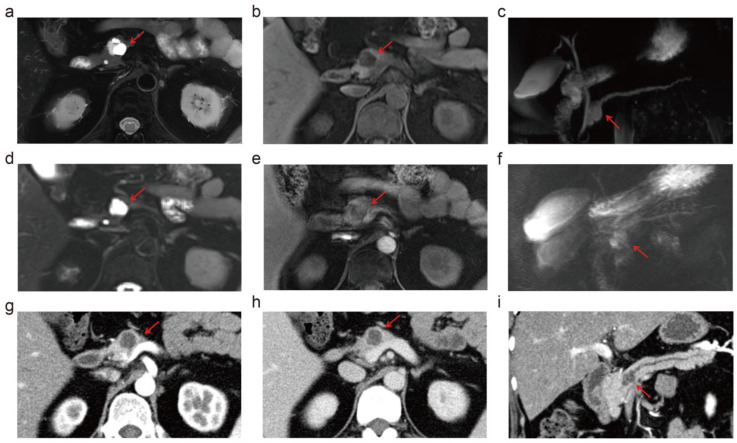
The images from 5.0 T MRI, 3.0 T MRI, and MDCT of a 56-year-old female. This patient was suspected of intraductal papillary mucinous neoplasm, which was stable at imaging follow-up. 5.0 T MRI demonstrates a sharper contour of the lesion and more delicate details, including the thin septa within the lesion and the communication between the cystic lesion and the mildly dilated main pancreatic duct (MPD), compared to the 3.0 T MRI. MDCT with curved planar reconstruction demonstrated the cyst–MPD communication as well but failed to show the thin septa within the lesion. (a–c) display the images from 5.0 T MRI of T2-weighted, T1-weighted, and MRCP, respectively. T2-weighted, T1-weighted, and MRCP images of 3.0 T MRI were attributed to (d–f), respectively. MDCT showed the lesions in arterial phase (g), portal venous phase (h), and curved planar reconstruction (i). The red arrows refer to the lesion.
